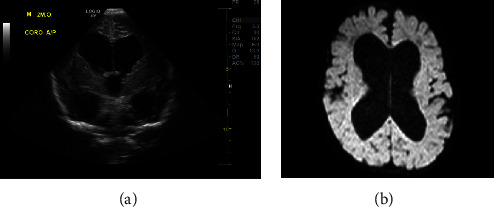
Head ultrasound (a) and MRI brain (b) showing a new finding of diffuse marked ventriculomegaly suggestive of communicating hydrocephalus.
